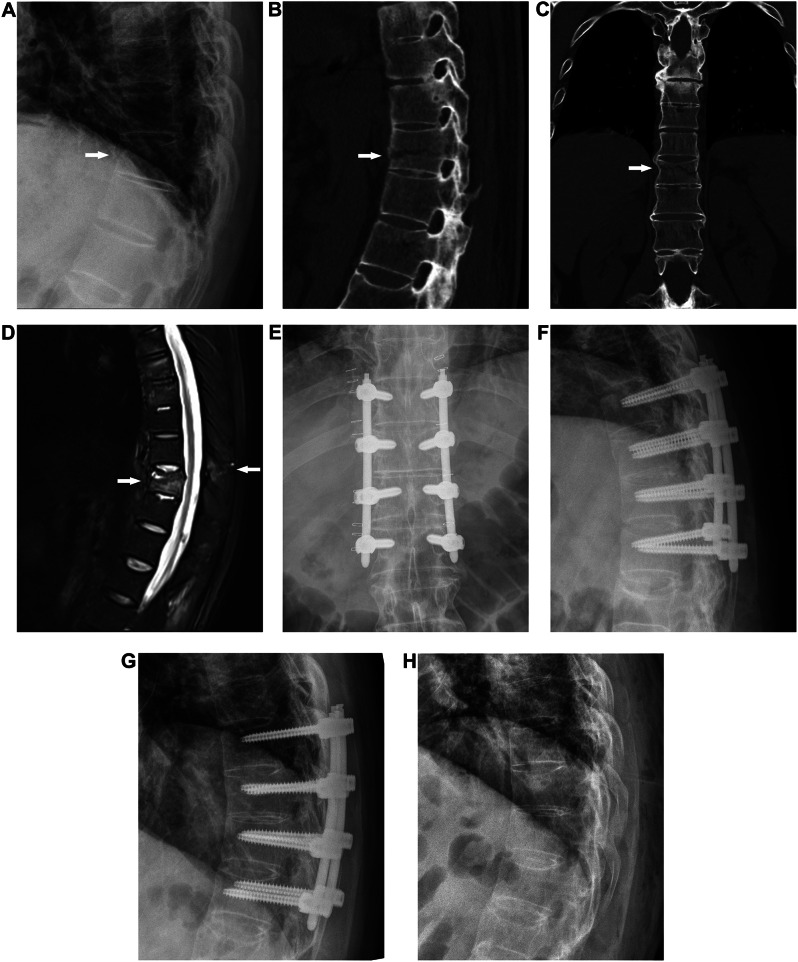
A 51-year-old man was diagnosed with a T11 fracture after sustaining an injury from a fall. Lateral radiography (A) reveals morphological changes in the T11 vertebral body (arrow). Preoperative computed tomography (B-C) demonstrated a fracture traversing the T11 vertebral body, pedicle, and articular process (arrow). Preoperative magnetic resonance imaging (D) showing a 3-column injury at T11 (arrow). Postoperative plain radiographs (E-F) demonstrate satisfactory screw placement. Lateral radiography (G-H) showed that the fracture had healed two years after the operation, and the internal fixation had been removed
